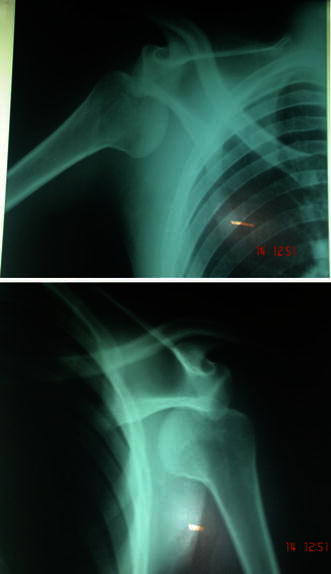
Radiographs of the shoulders demonstrate bilateral anterior dislocation
